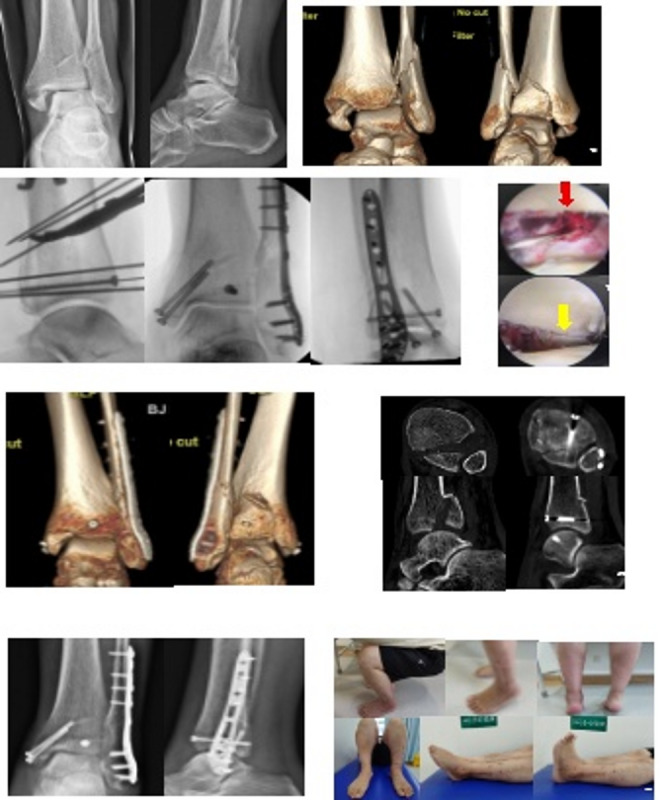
A 42-year-old male with a left trimalleolar fracture (AO/OTA 44-C2.3, Bartoníček Type IV) following a traffic accident. Preoperative imaging confirmed the diagnosis a, b. Intraoperative fluoroscopy showed satisfactory reduction with congruent joint surfaces (Congruity, green) and stable syndesmosis (Syndesmosis, green) without fixation c. Arthroscopy revealed pre-reduction articular step-off (red arrow) and post-reduction restoration (< 1 mm step/gap, green arrows), yielding an “All-Green” SGSC rating d. Postoperative CT confirmed these findings e, f. At 12 months, radiographs demonstrated solid union without arthritis (g), with an AOFAS score of 90 h
